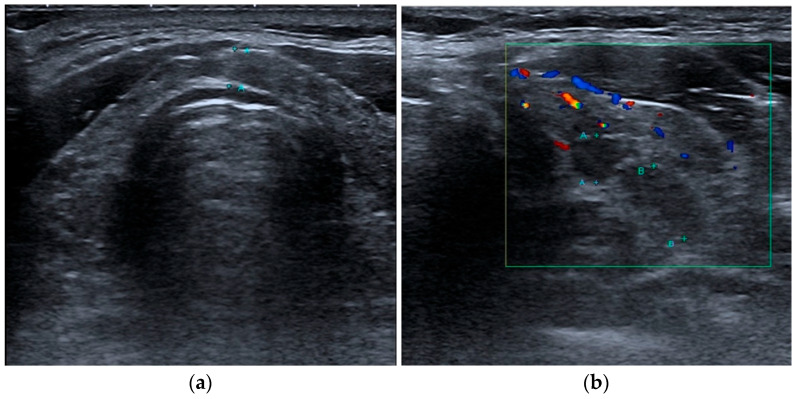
Thyroid ultrasound examination results after MOK pharmacopuncture treatment: The isthmus thickness was slightly reduced (a), the size of the pre-existing cysts in the left thyroid decreased, and slight parenchymal changes were observed in both thyroids, indicating an improvement in thyroiditis (b). A: 4.4mm distance, B: 7.5mm distance.
